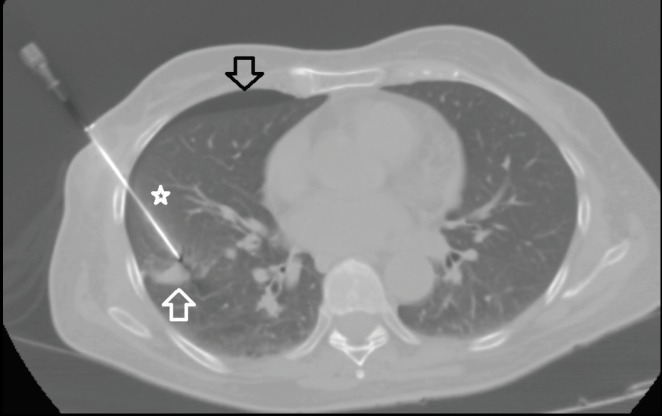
CT-guided lung biopsy of right sided 24 mm pulmonary
